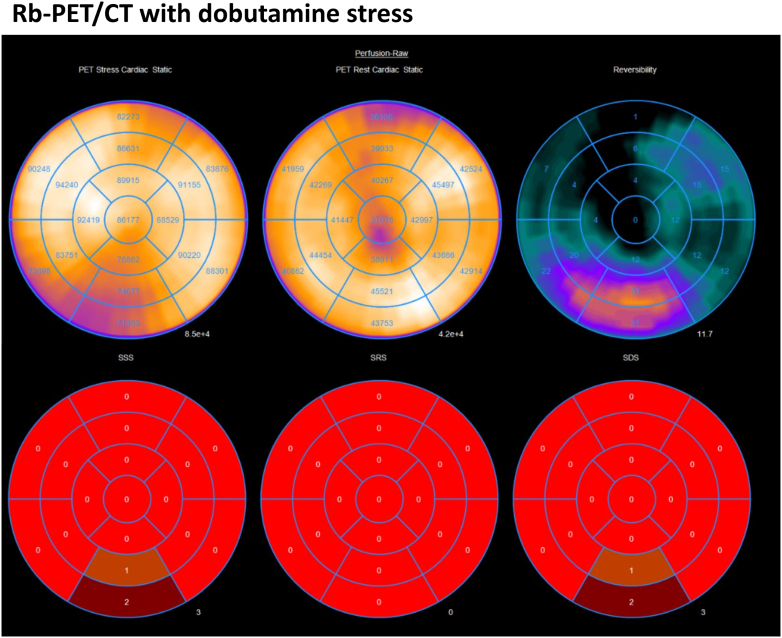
Rb-PET/CT During Dobutamine Stress (Left) Rb-PET/CT stress perfusion map during dobutamine-atropine volume challenge showing a reversible perfusion defect in the inferior myocardium (AHA segments 4 and 9), corresponding to ∼5% of left ventricular mass. (Right) Matched rest perfusion demonstrating full reversibility. Images are shown in short-axis orientation with the same intensity scale. AHA = American Heart Association; Rb-PET/CT = rubidium positron emission tomography/computed tomography.
